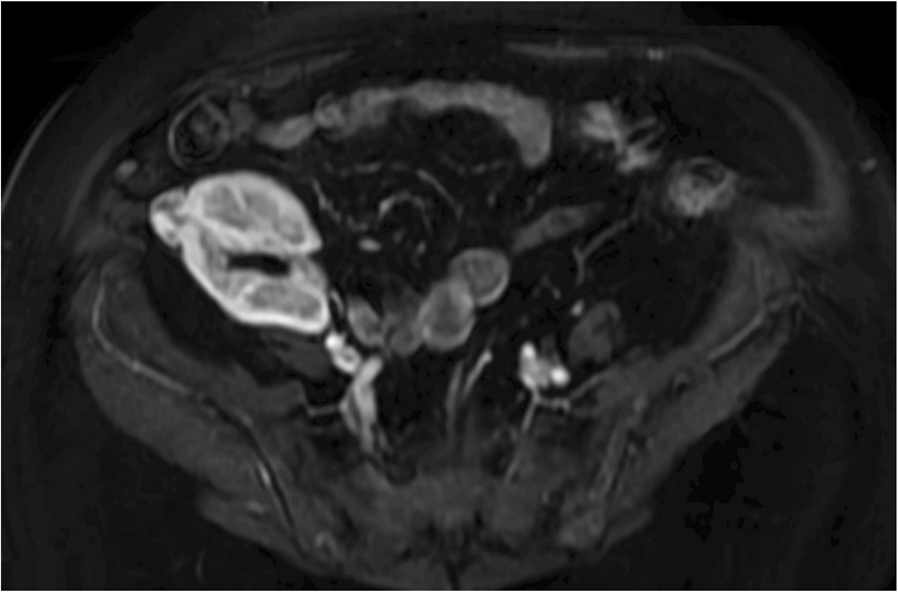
Magnetic resonance imaging (MRI) was used for the follow-up evaluation of renal mass because of its multi-planar capabilities, as well as its ability to demonstrate of enhancement and to provide soft-tissue contrast. A MRI scan at 60 months after transplantation showed a well-circumscribed oncocytoma with expansive net margins at the cortical surrounding of the kidney (white arrows). It has not infiltrative margins with extension in peri-renal fat or renal sinus, in the medulla of the kidney or in the major renal venous vessels
